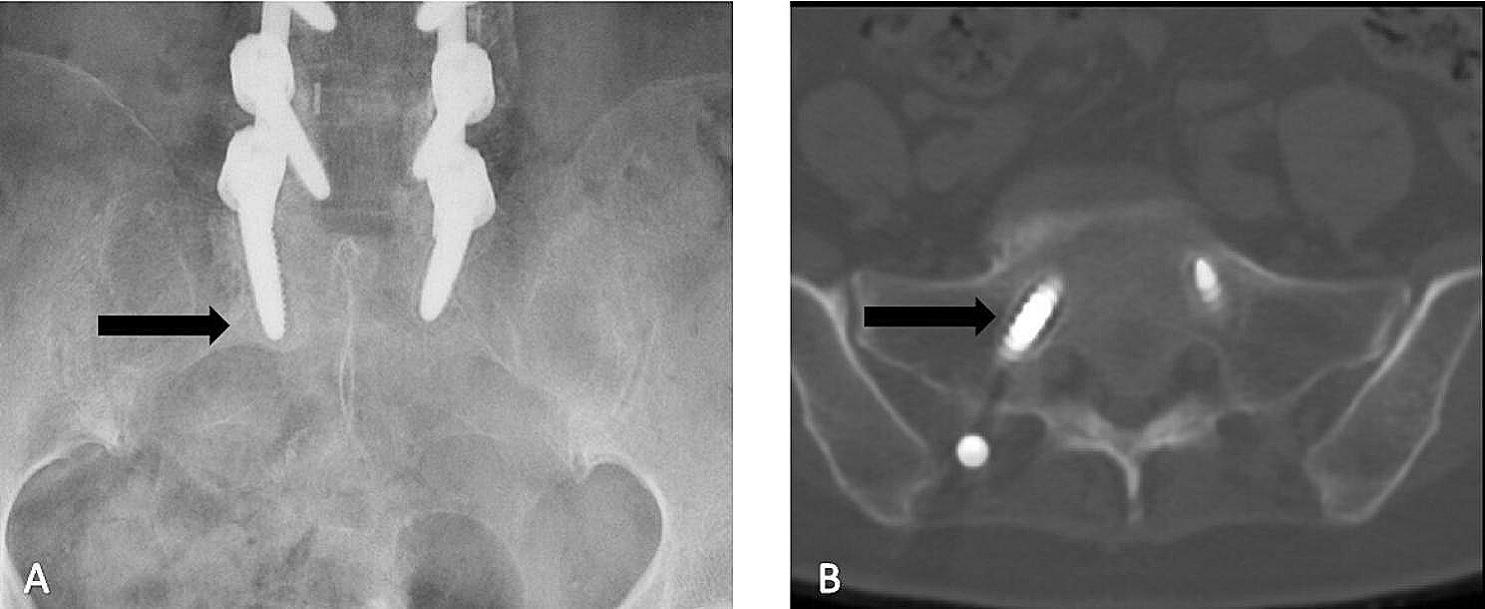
A halo sign surrounding the first sacral vertebra (S1) pedicle screw on radiographs. (A) The radiolucent line on a plain radiograph is indicated by a black arrow. (B) The radiolucent line on the computed tomography image is indicated by a black arrow
